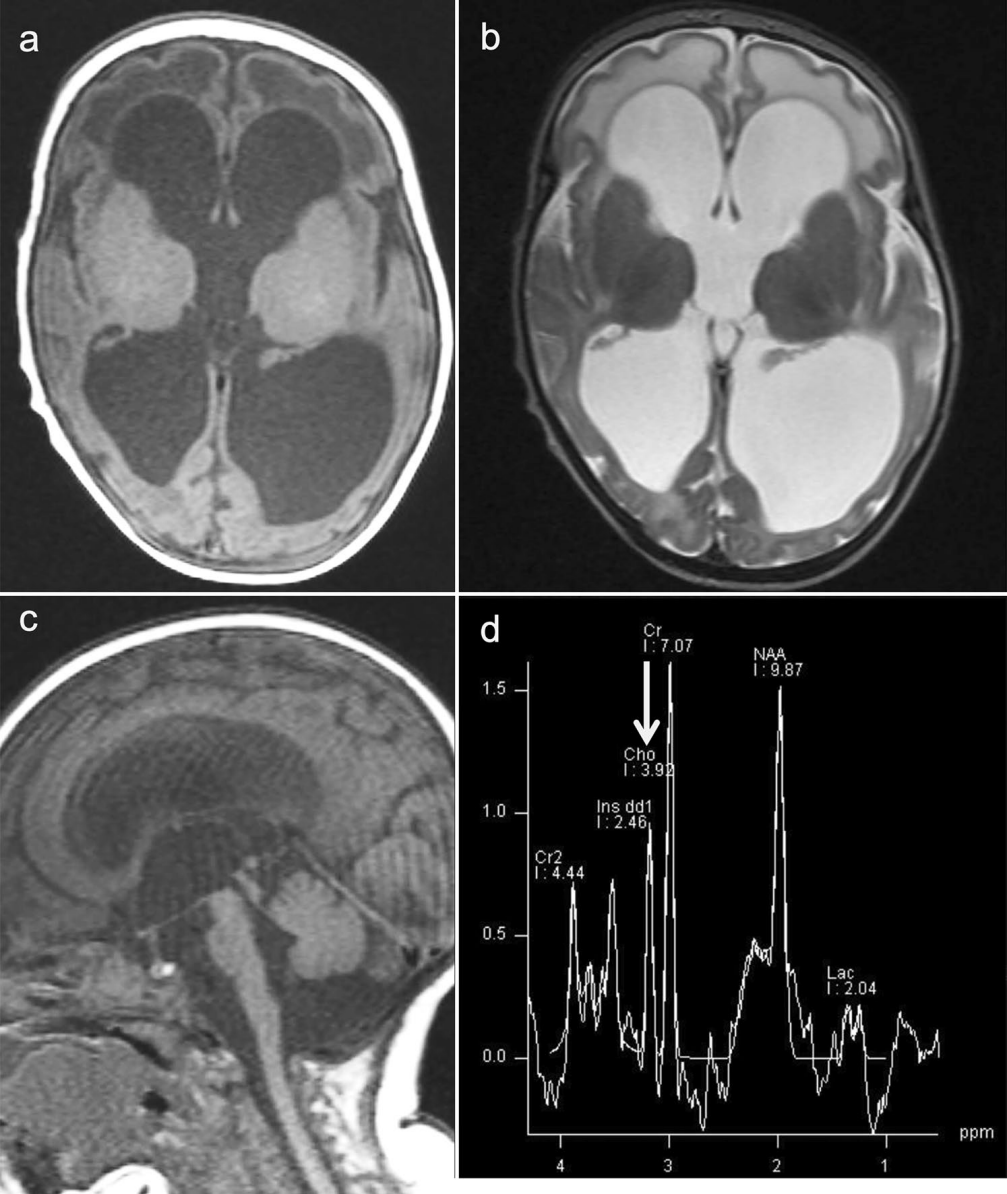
A 2-month-old, female patient with 5,10-methylene-tetrahydrofolate reductase deficiency (MTHFRD). a Ax T1-weighted images demonstrated marked dilatation of all ventricles indicating communicating hydrocephalus. b T2-weighted images demonstrated hyperintensity in the bilateral frontal periventricular white matter. Delayed myelination can also be seen. c Sagittal T1-weighted images demonstrated mild hypoplasia of the pons and cerebellum. d ^1^H-MRS (TE = 30) demonstrated a slightly low cho (arrow) and NAA peak (Source: reference 53)
